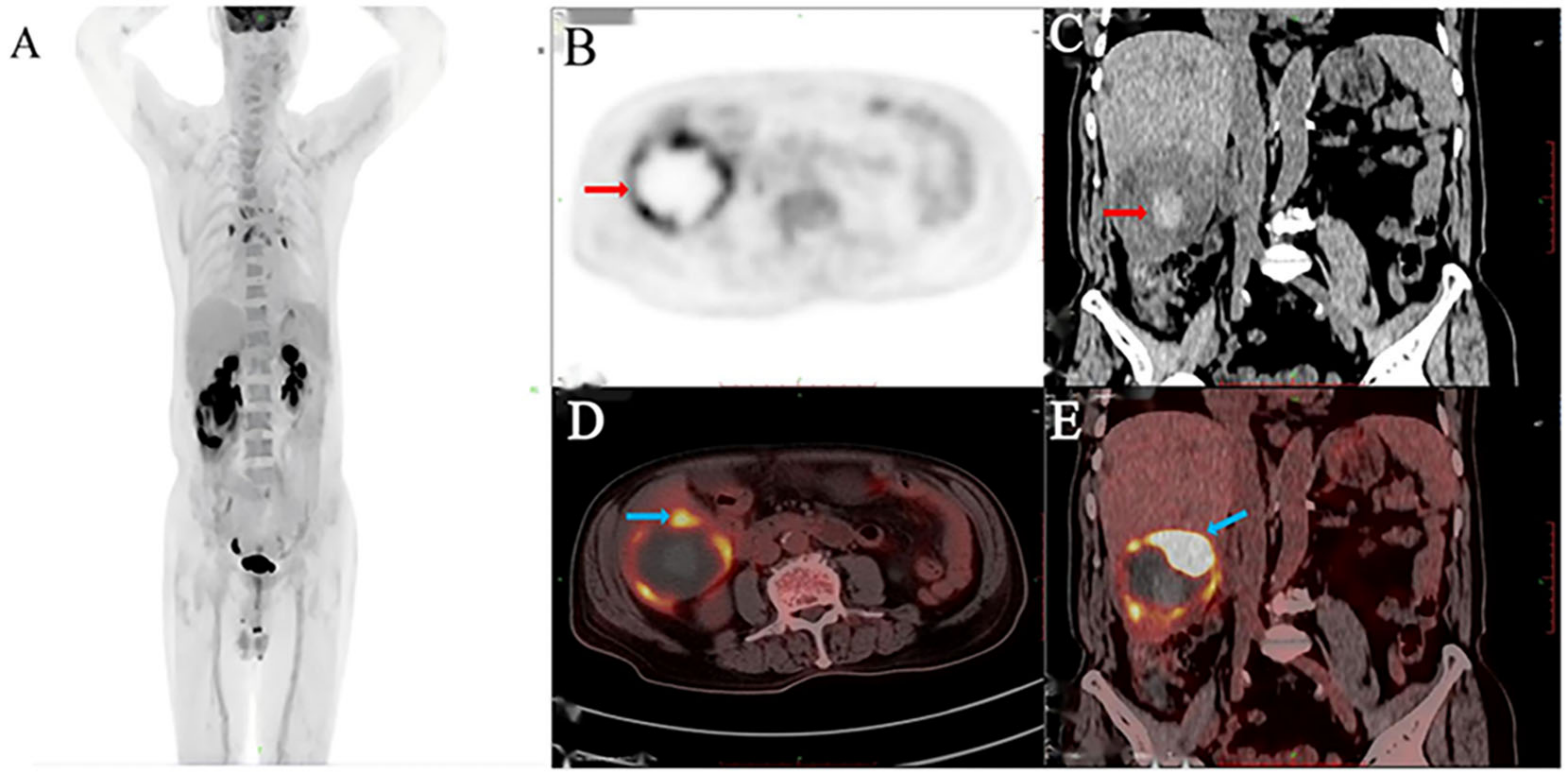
18F-FDG PET/CT imaging identified a hepatic malignancy. The MIP image (A), PET (B), CT (C), and axial (D) and coronal (E) fused images revealed a hypermetabolic right hepatic tumor with an SUVmax of 21.4.
